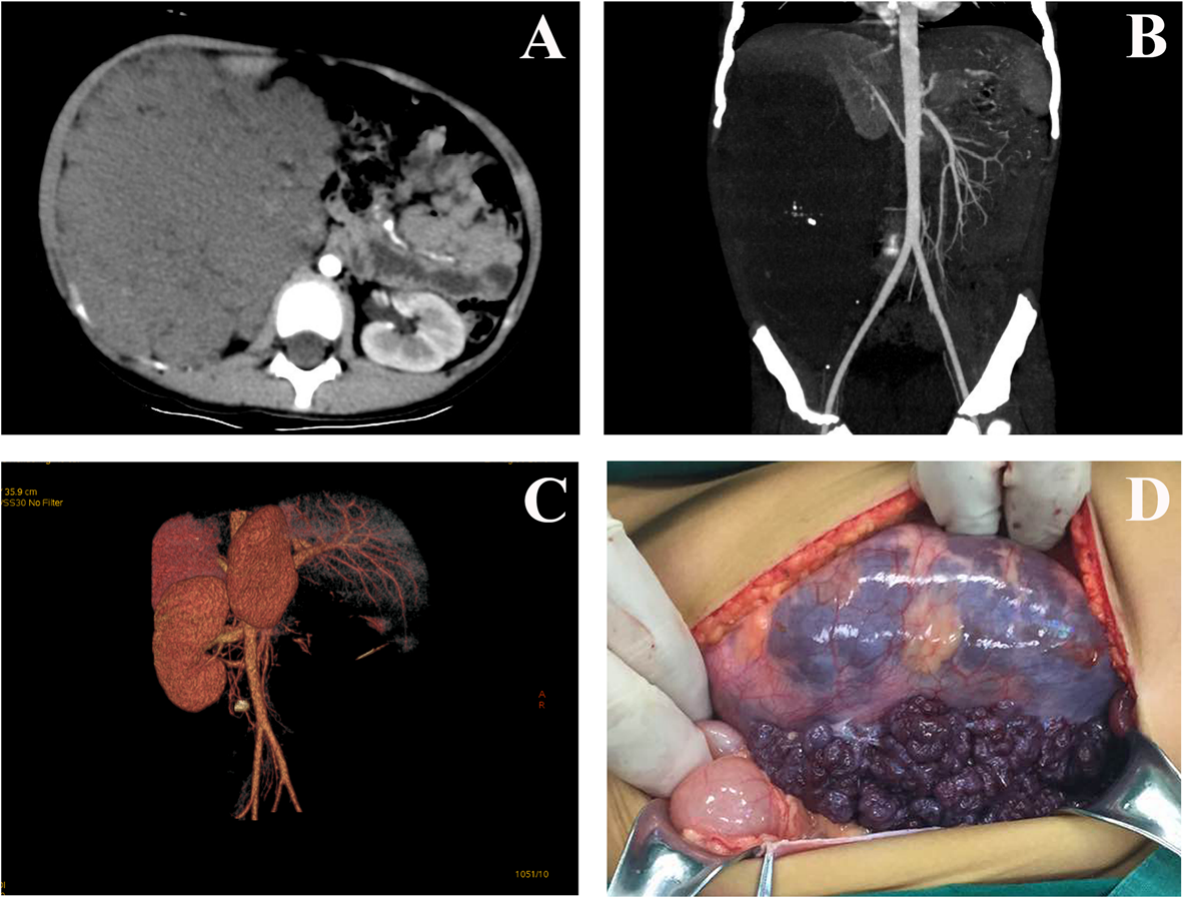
a: CT scan showed a large soft tissue mass in the right retroperitoneum, about 10 cm × 13 cm × 17 cm in size, and contrast-enhanced CT scan showed no obvious enhancement inside the mass. b: CT scan showed multiple phleboliths formed in the lesion, and the blood supplying vessels was invisible in the mass. c: Three-dimensional reconstruction of CTA did not show image of the right retroperitoneal mass, and the blood supplying in mass was not seen, either. d: In the operation, the giant soft tissue mass in the right peritoneum was venous malformation. The color of the mass was dark purple. Lesion blood vessels were flexion and expansion. The mass capsule was very thin and was prone to rupture and bleeding
